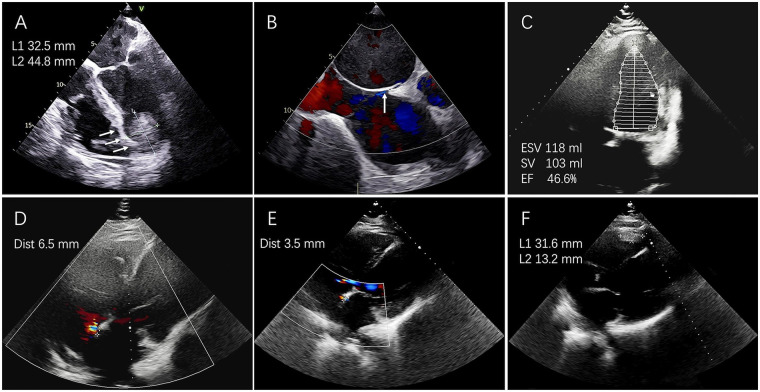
Follow-up echocardiograms. (A) Pre-procedural echocardiogram showing a large protruding, circular thrombus in the left ventricular apical aneurysm (arrow). (B) Transesophageal echocardiogram showing a patent foramen ovale (PFO) with a left-to-right shunt (arrow). (C) Left ventricular ejection fraction 1 month after the procedure. (D) Transthoracic echocardiogram showing a PFO of about 6.5 mm diameter 1 month after the procedure. (E) PFO in March 2024. (F) Mural thrombus in March 2024.
